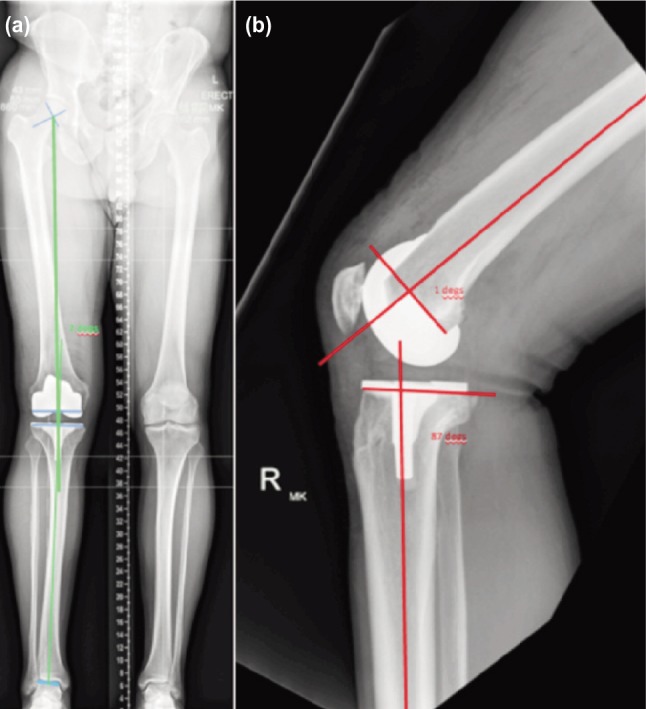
(a) Post-operative measurements of Hip-Knee Angle on 4-foot standing films and (b) Sagittal Femoral Angle and Sagittal Tibial Angle on 18-inch long lateral radiographs.
